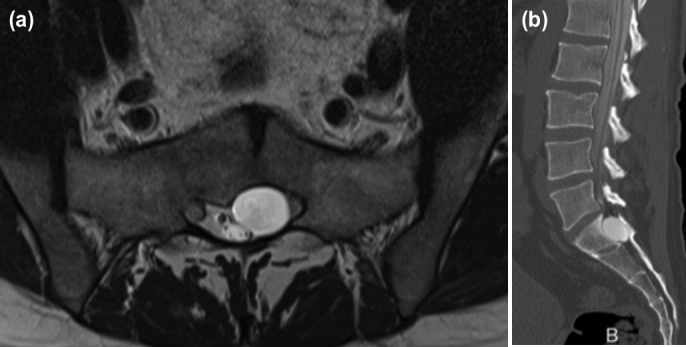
(a) MRI axial view and (b) CT sagittal view showing location of arachnoid cyst in sacral canal in close proximity with left S1 nerve root.
